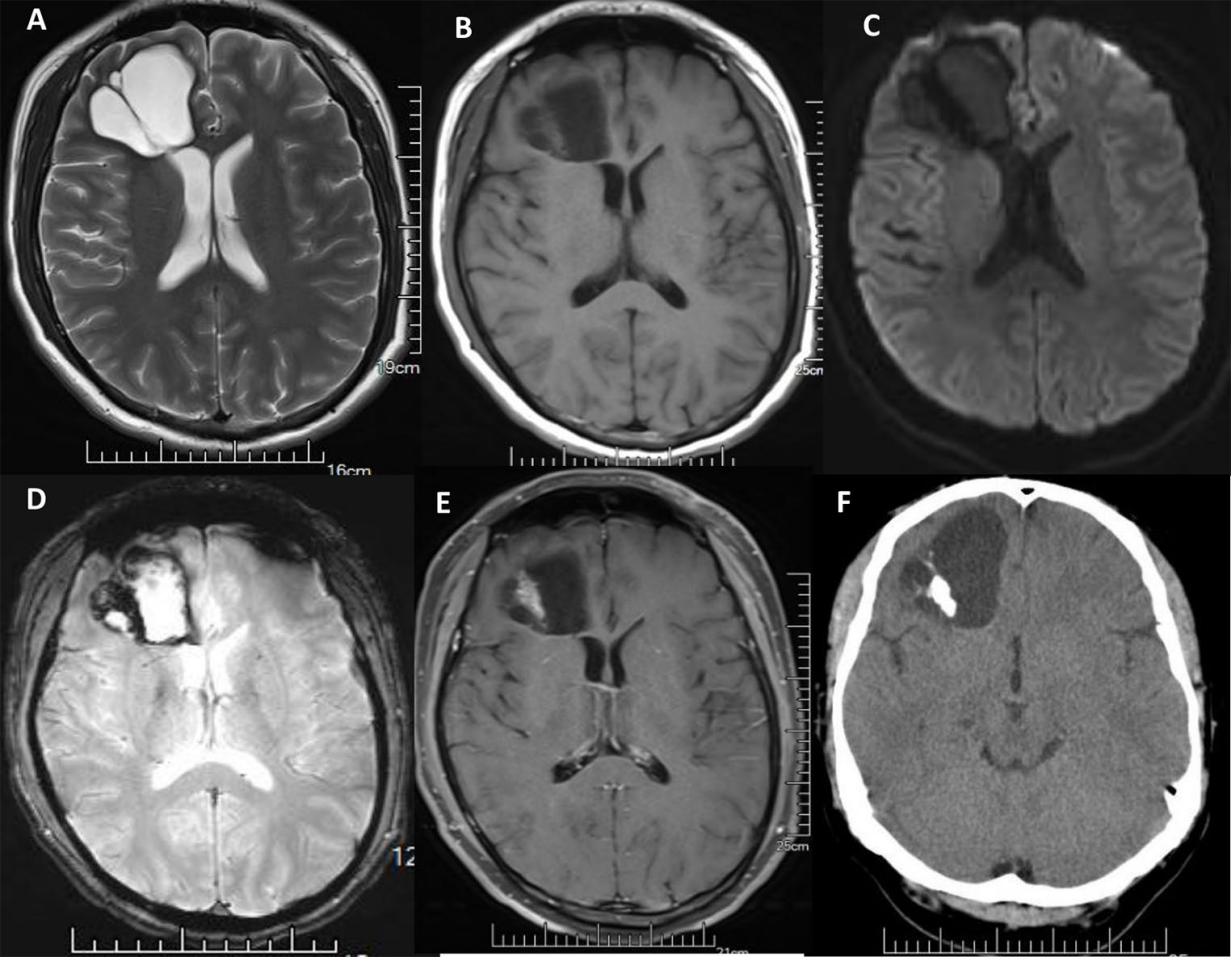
PGNT in the right frontal lobe of a 38-year-old male. A. Axial T2WI shows separation signals within PGNT. B. Axial T1WI shows mixed cystic and solid lesion. C. No diffusion restriction is observed in axial DWI. D: Axial SWI shows calcifications and hemorrhagic signals within the lesion. E. Axial enhancement shows heterogeneous enhancement in the solid part of the lesion. F. Axial CT shows spotty and patchy calcification within the lesion.
